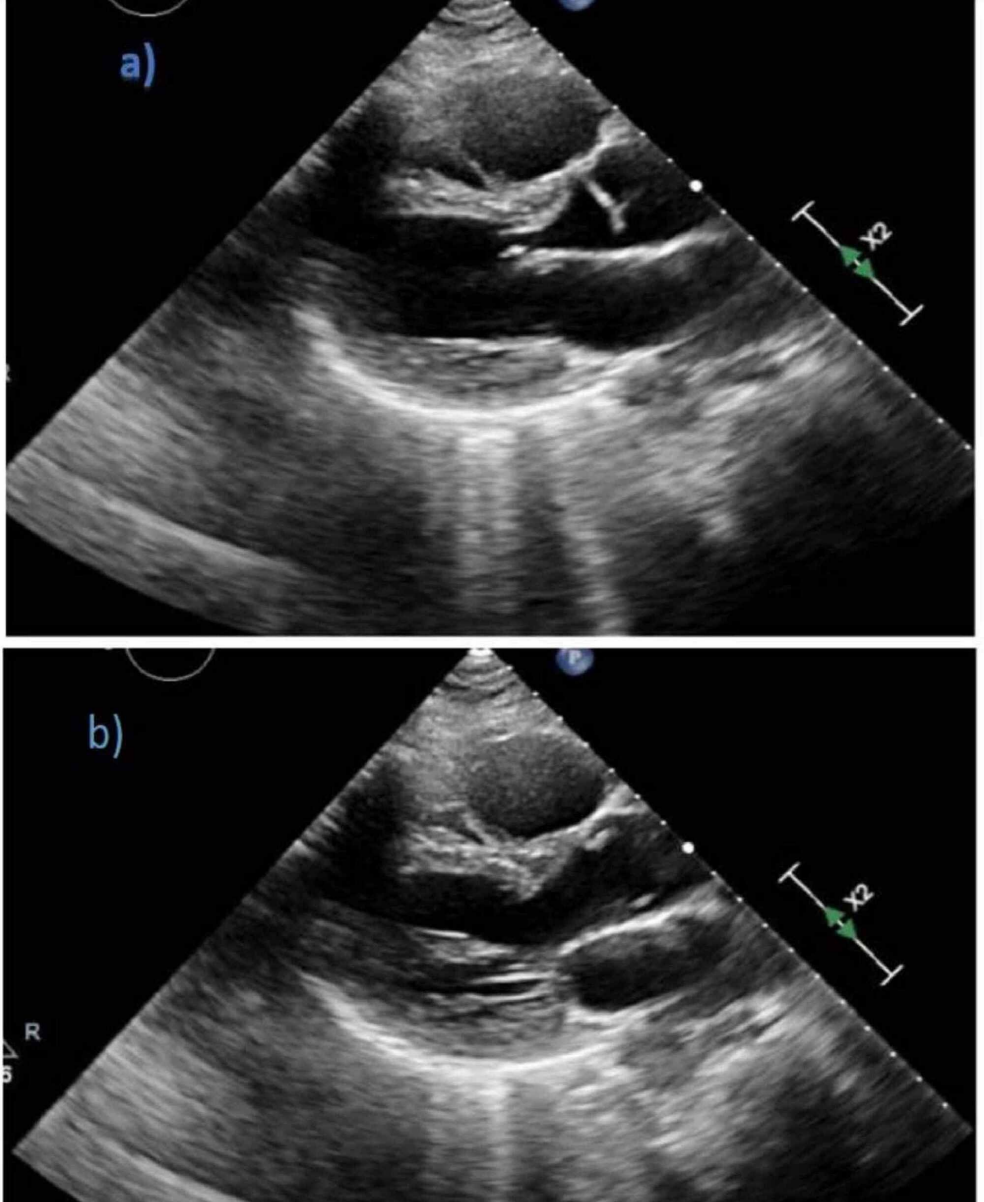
Transthoracic echocardiogram showing global hypokinesis of the left ventricle with an estimated ejection fraction (EF) of 25% during diastole (a) and systole (b) on parasternal long-axis view
